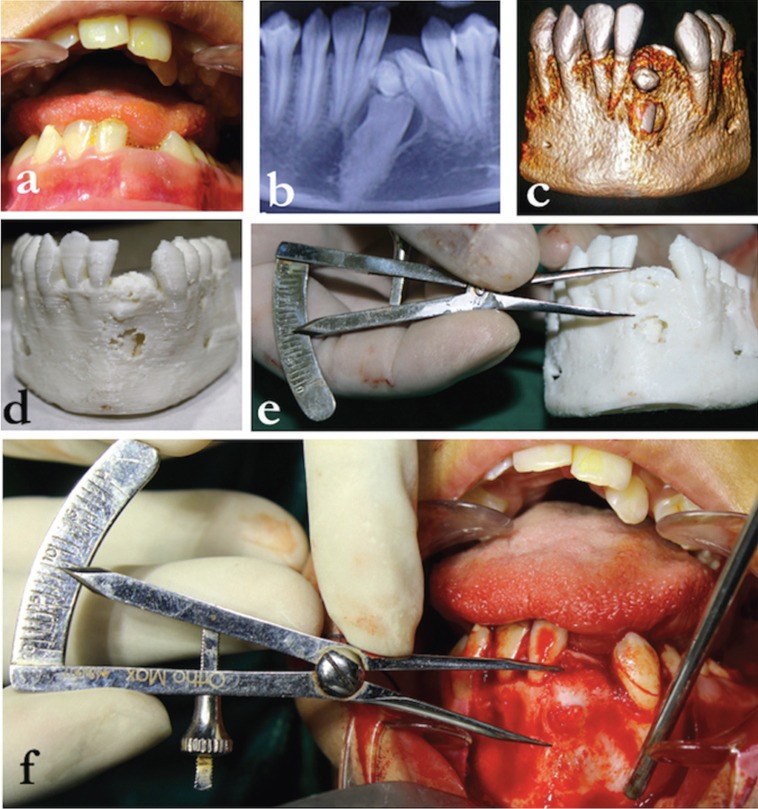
a) Anterior view showing absence of 31 and 32. b) CBCT scan showing cystic involvement with relation to impacted 31, 32 and an odontome. c) CBCT scan showing the presence of impacted 31, 32 and an odontome. d) 3D printed model of the mandible. e) Pre-surgical measurements being made on the 3D printed model. f) Transfer of pre surgical measurements on to the surgical area.
